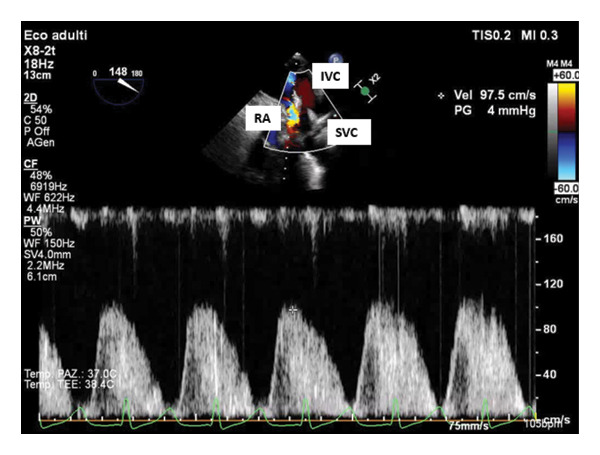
Deep transgastric pulsed wave color Doppler flow velocity after releasing the superior vena cava suture. RA: right atrium; IVC: inferior vena cava; SVC: superior vena cava.
